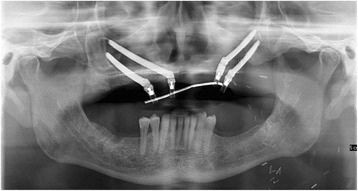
Panoramic dental radiograph showing the position of the zygomatic implants and the seating of the initial fixed prosthesis
